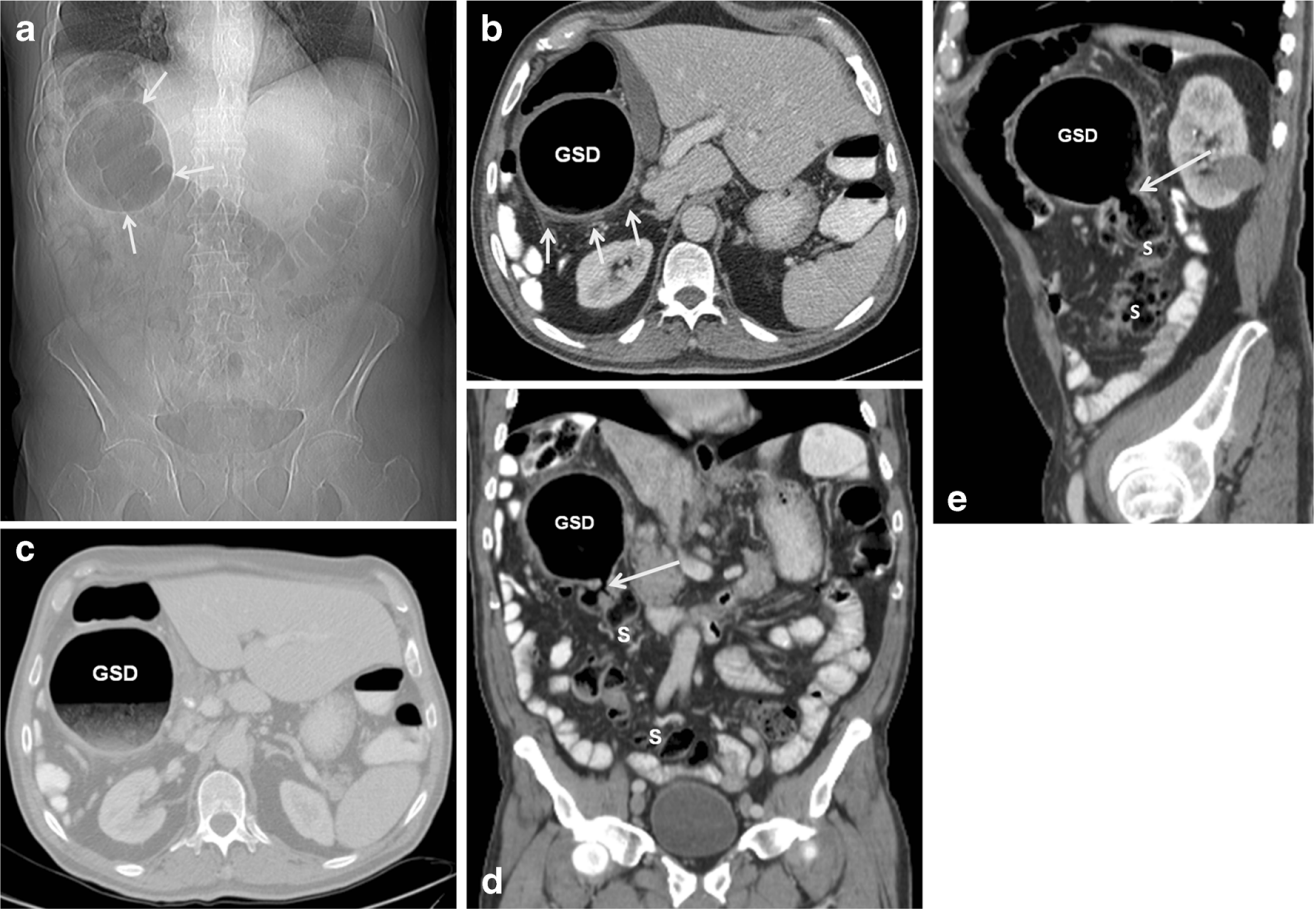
A 55-year-old man with giant sigmoid diverticulum (GSD) associated with acute diverticulitis. Abdominal radiograph (a) shows a large, round, homogenous radiolucency in the right upper quadrant that is smoothly marginated (arrows). Axial (b and c), coronal (d), and sagittal (e) contrast-enhanced CT images through the upper abdomen show a predominantly gas-filled structure in the right upper abdomen, communicating with the sigmoid colon (S) and consistent with GSD. The sigmoid colon is located in the right side of the abdomen (anatomical variant). The arrow demonstrates the neck of the GSD, which connects the diverticular cavity with the adjacent sigmoid colon; this finding is essential for correct diagnosis. The thickened wall of the diverticulum and the surrounding mesentery infiltration denote acute diverticulitis
